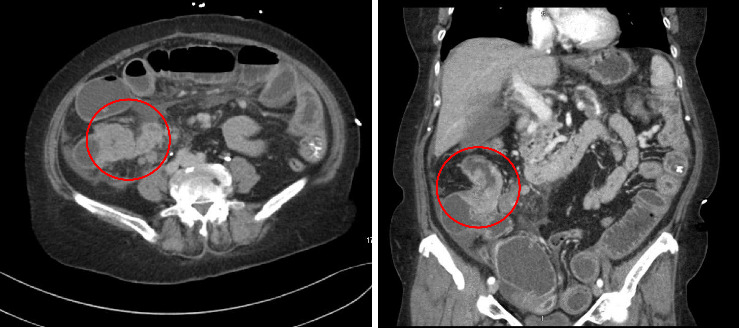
CT abdomen/pelvis with contrast showing ascending colon obstructing mass.
